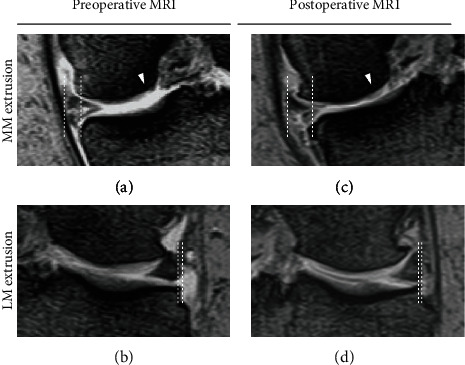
Magnetic resonance imaging—coronal view. (a) Preoperative medial meniscus extrusion (MME) (4.3 mm) and highly damaged articular cartilage on the medial femoral condyle (arrowhead). (b) Preoperative lateral meniscus extrusion (LME) (1.2 mm). (c) Postoperative MME (7.0 mm) and improved articular cartilage (arrowhead). (d) Postoperative LME (0.9 mm).
